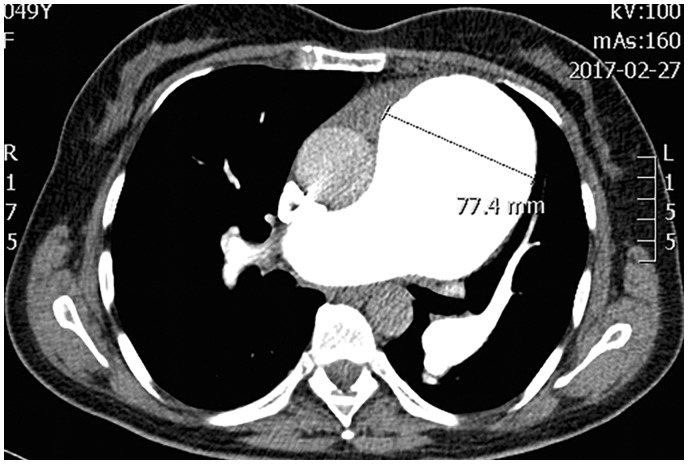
CT scan demonstrating further dilatation of the pulmonary artery to 77 mm.
